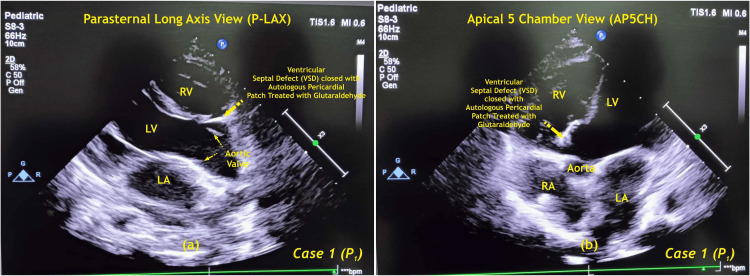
Postoperative 2D echocardiography of case one (P1) RA - right atrium; LA - left atrium; RV - right ventricle; LV - left ventricle; P - patient Image Credits: Dr. Vishal V. Bhende
